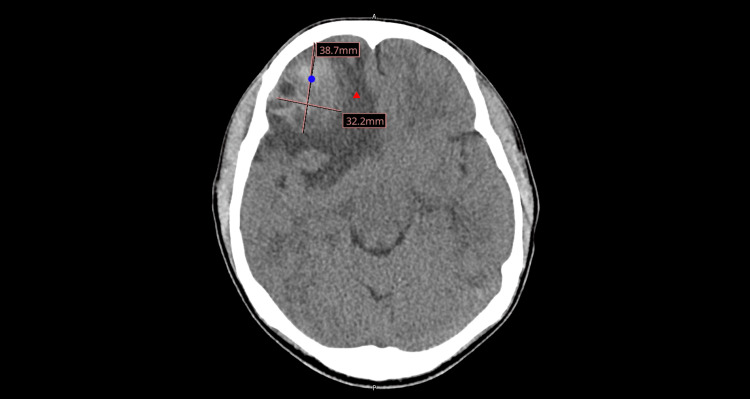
CT scan showing right frontal hemorrhage (blue circle) with vasogenic edema (red triangle) and midline shift.
